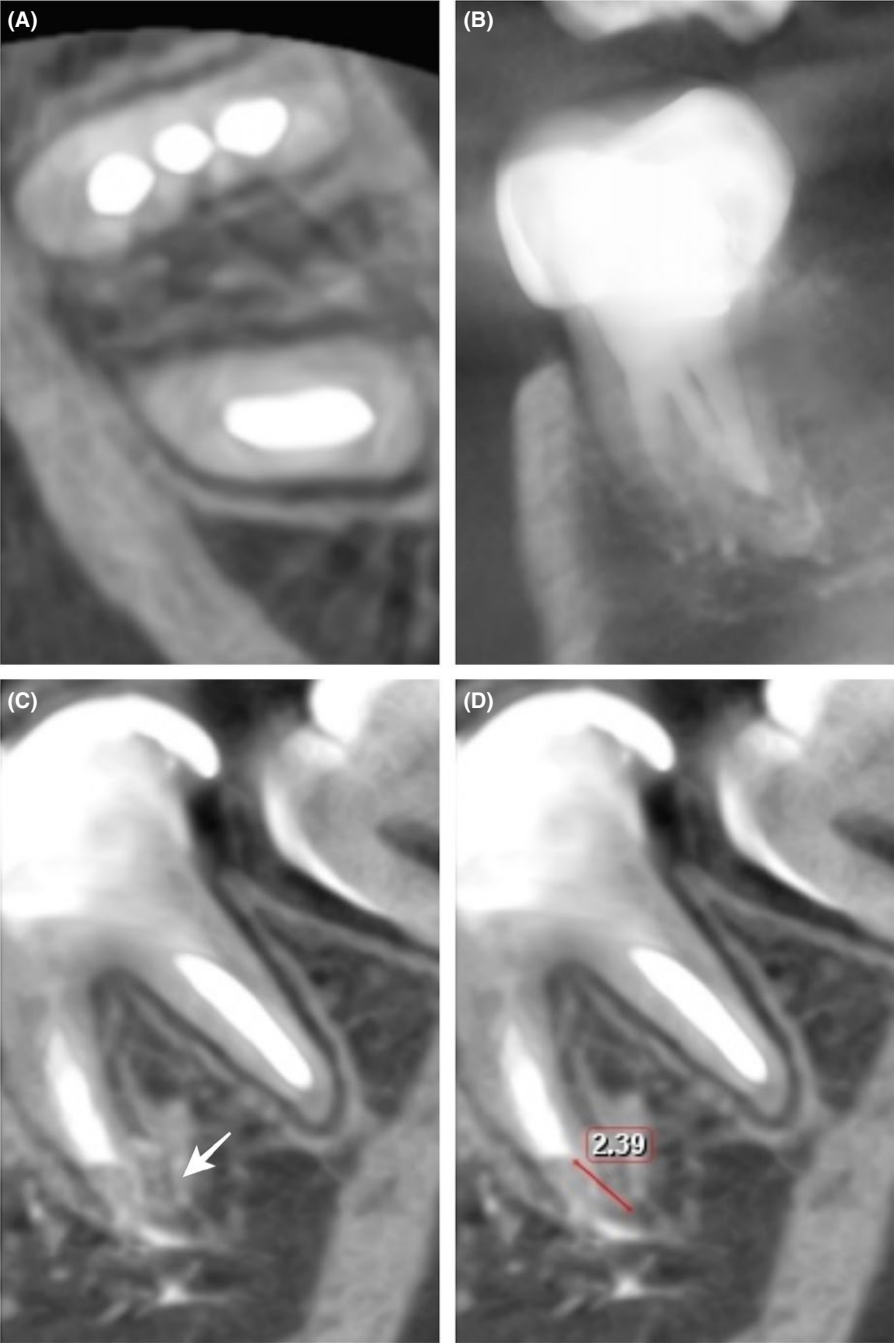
Cone beam computed tomography scan at 24 months. A and B, Obturation of three mesial canals and (C) continued root development (arrow) are observed. D, An approximate measure was obtained from the end of the mineral trioxide aggregate barrier to the end of the root apex
